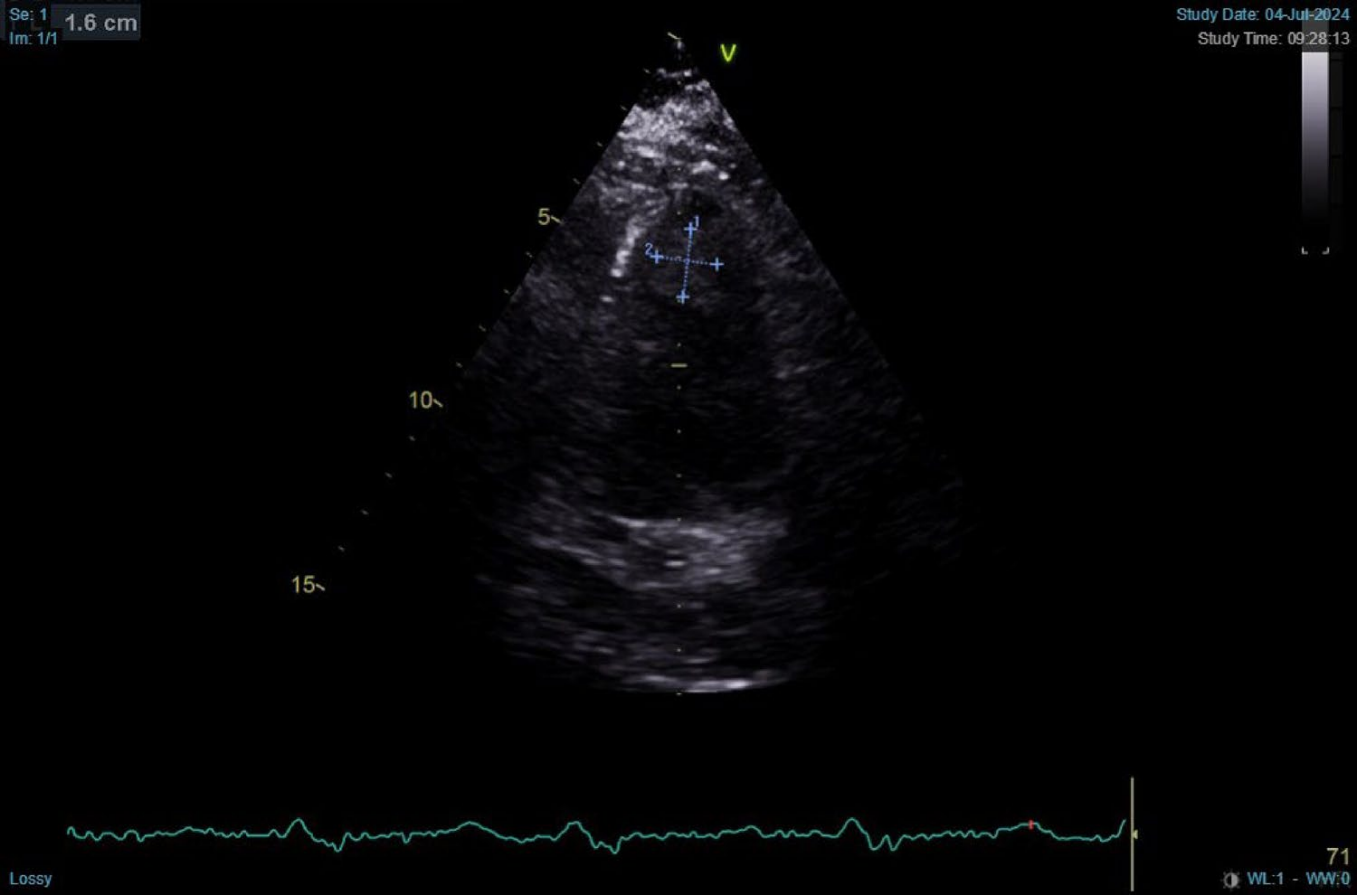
Patient’s echocardiography shows intracardiac thrombus
